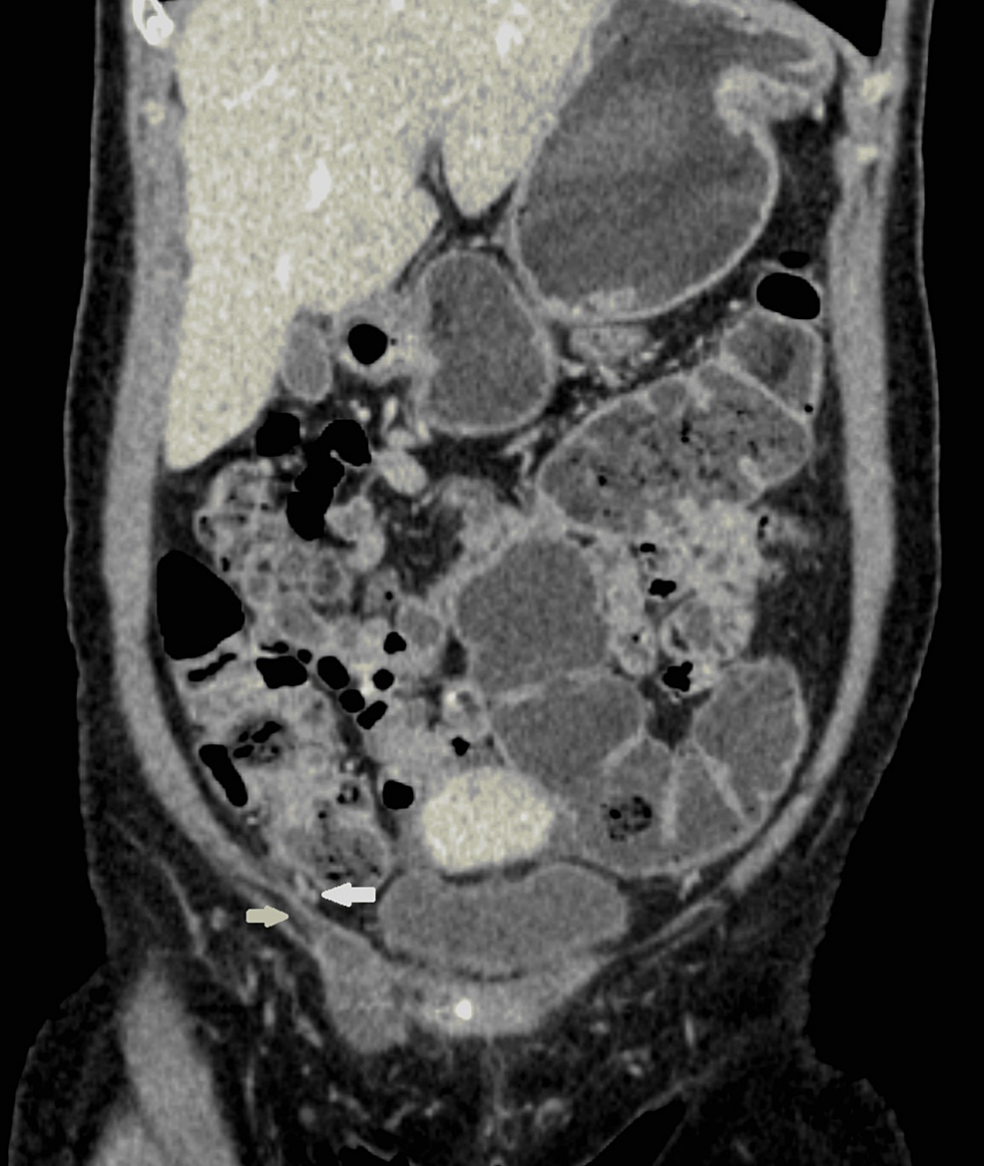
Coronal CT scan of authors' patient in venous phase shows the proximal origin of hydrocele of the canal of Nuck (yellow arrow) lateral to inferior epigastric vessels (white arrow)
